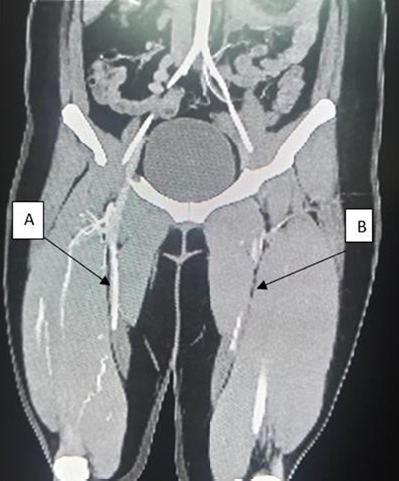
CT angiography of the bilateral lower limb (coronal view) shows significant narrowing from the middle to the distal segments of the bilateral superficial femoral arteries, worse on the left femoral artery. (A) Right femoral artery. (B) Left femoral artery.
